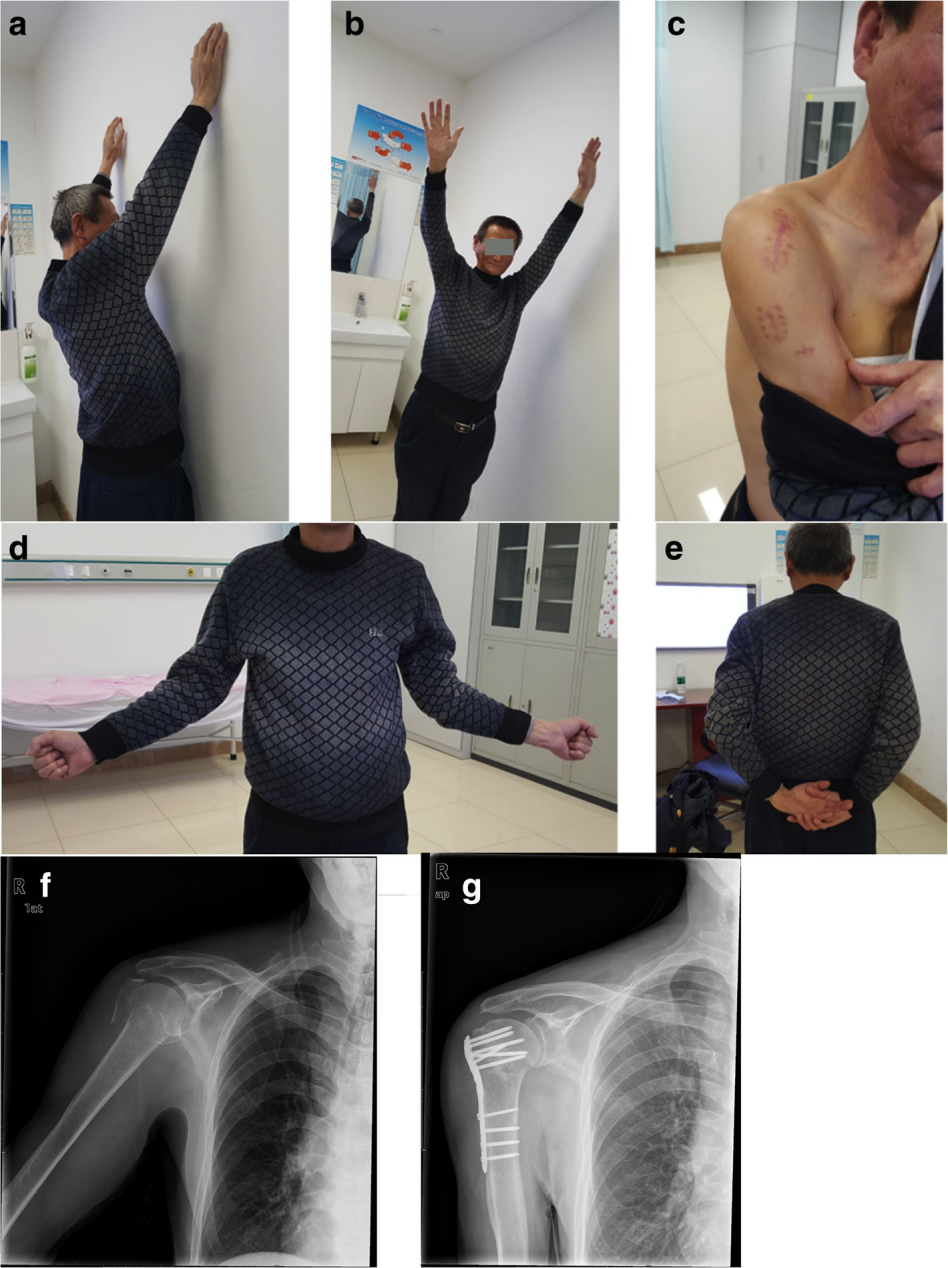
Clinical and functional outcome. a–e Function at the latest follow-up. f Preoperative X-ray. g Post-operative X-ray
